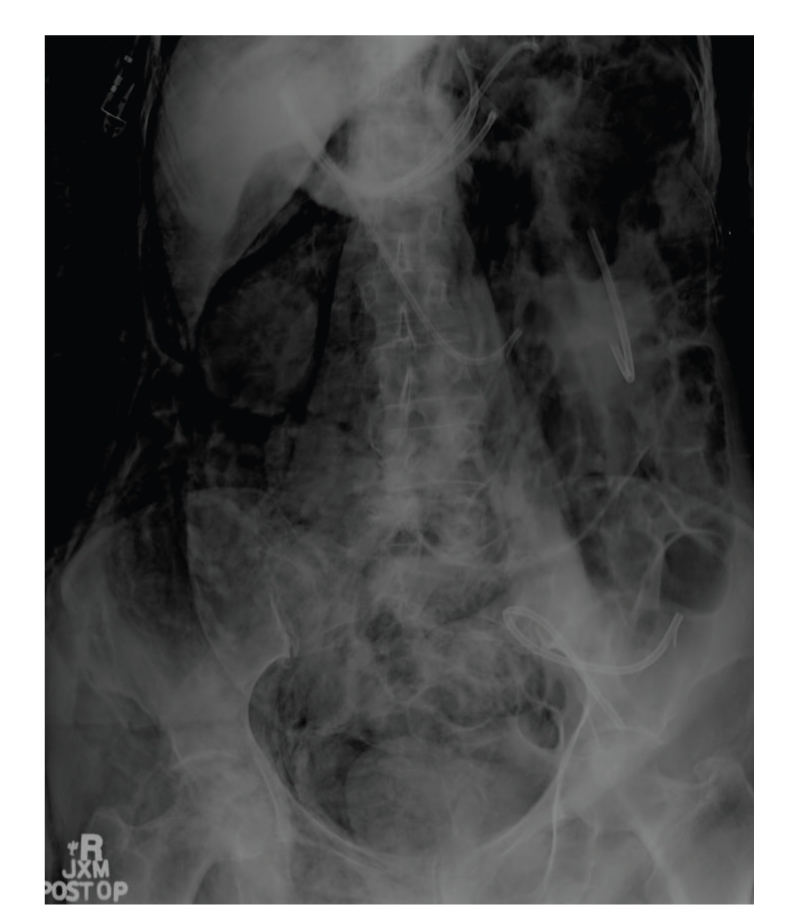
Portable abdominal X-ray showing retroperitoneal and intraperitoneal-free air.
